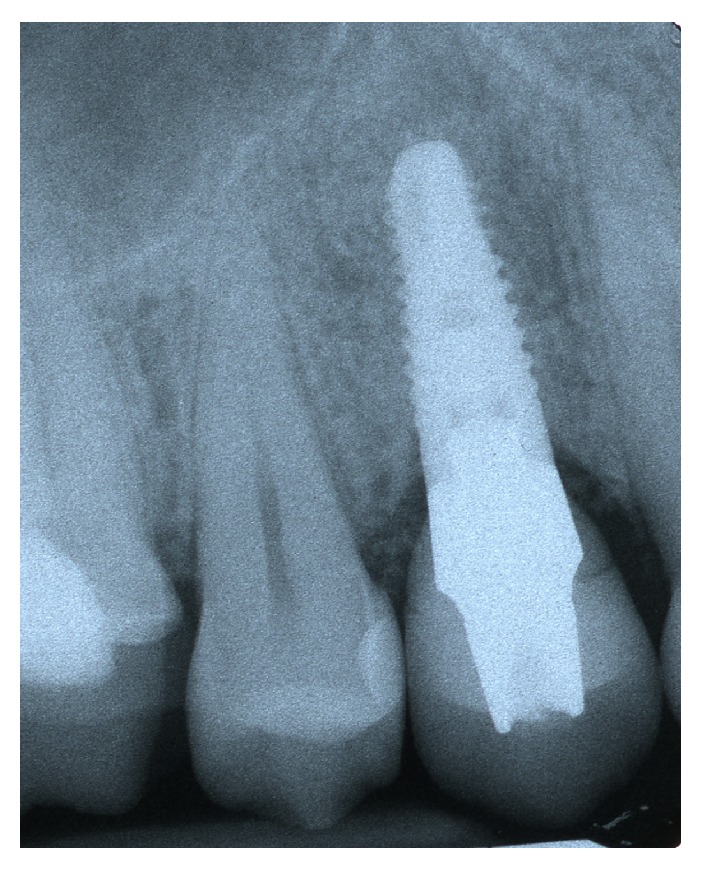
The periapical radiograph of the implant and the cement restoration (two pieces) 36 months after implant loading. Almost no edgeal bone loss was noted around the implant.
